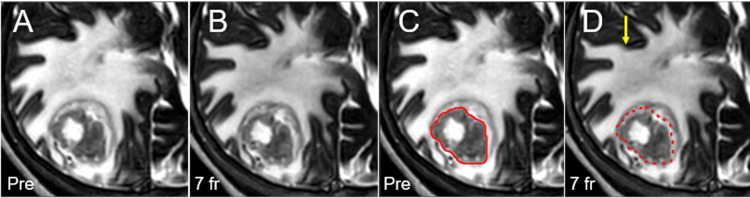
Magnetic resonance images obtained at one day before and at completion of stereotactic radiosurgery. Images show axial T2-WI (A-D); before SRS (A, C); at completion of SRS (7 fr) (B, D); the GTV contoured on the planning T2-WI (C); and the GTV contour (dashed) superimposed on the T2-WI at 7 fr. (A-D) All images were co-registered and are shown in the same magnification and coordinates. (B, D) At completion of 7 fr SRS, a slight dorsal displacement of the GTV without significant volume change is observed due to slight mitigation of the peritumoral edema (arrow in D). WI: weighted image; SRS: stereotactic radiosurgery; fr: fraction; GTV: gross tumor volume
